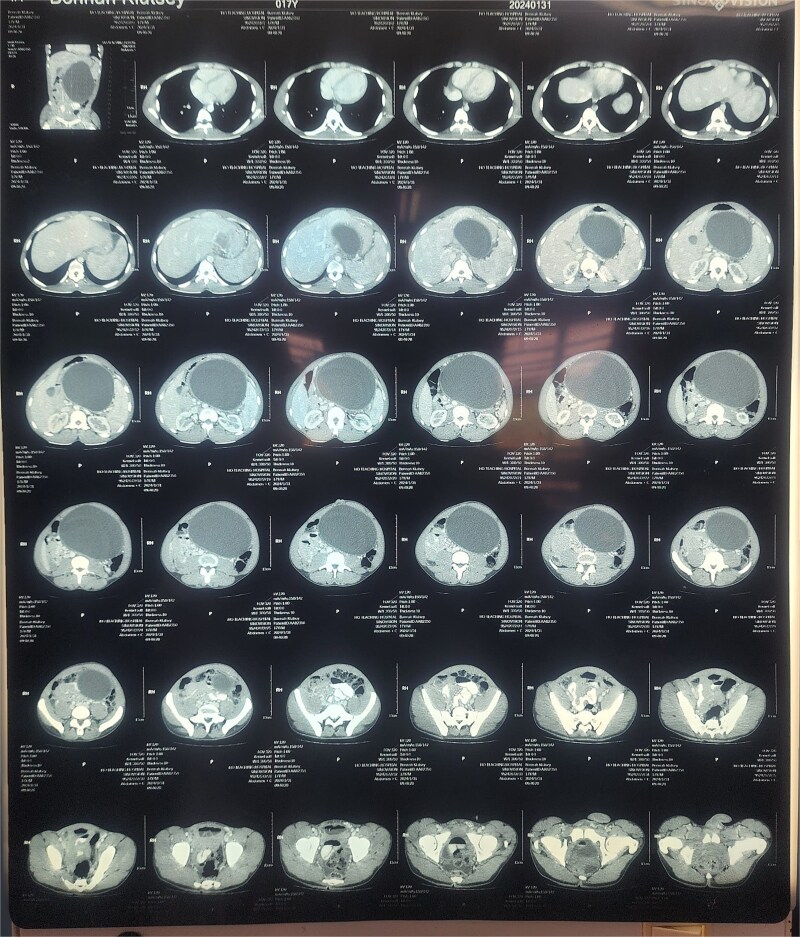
Contrast-enhanced CT scan of the abdomen demonstrating an 18.5 × 10.0 × 13.5 cm well-circumscribed, unilocular pancreatic cyst lesion in the region of the body and tail of the pancreas.
